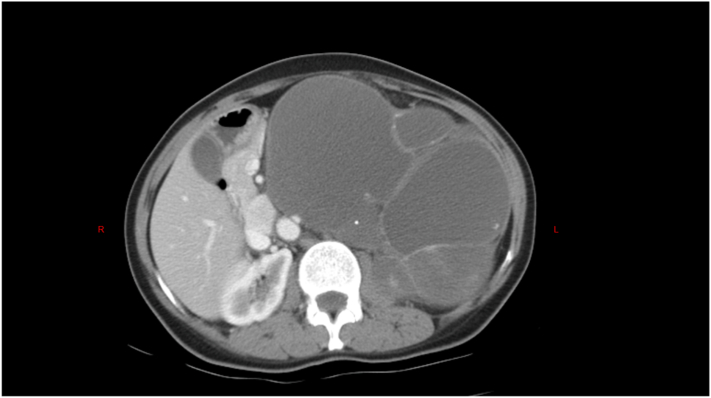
Computed tomography (CT) image of the large hypoechoic abdominal mass with cystic components posterior to the stomach and pancreas extending up to the left subdiaphragmatic and splenorenal spaces.
